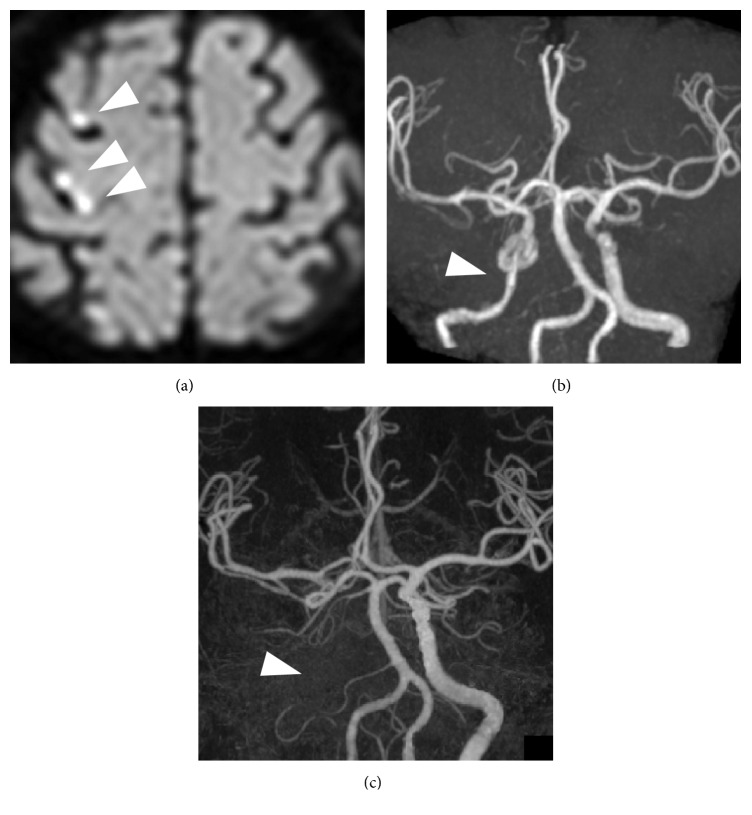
Diffusion-weighted MRI taken on the day of onset indicated multiple infarcts in the right cerebral cortex (a, arrowheads). MR angiography performed on the same day revealed blood flow in the internal carotid artery (b, arrowhead), but CT-angiography conducted 7 days after onset of the disease showed complete occlusion of the artery.
